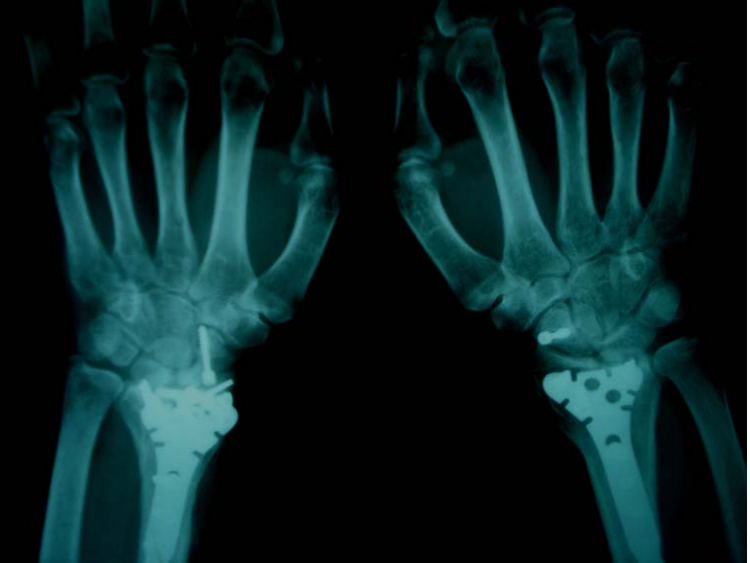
Postoperative AP roentgenogram of the right and left distal radius and scaphoid at 3 months.
